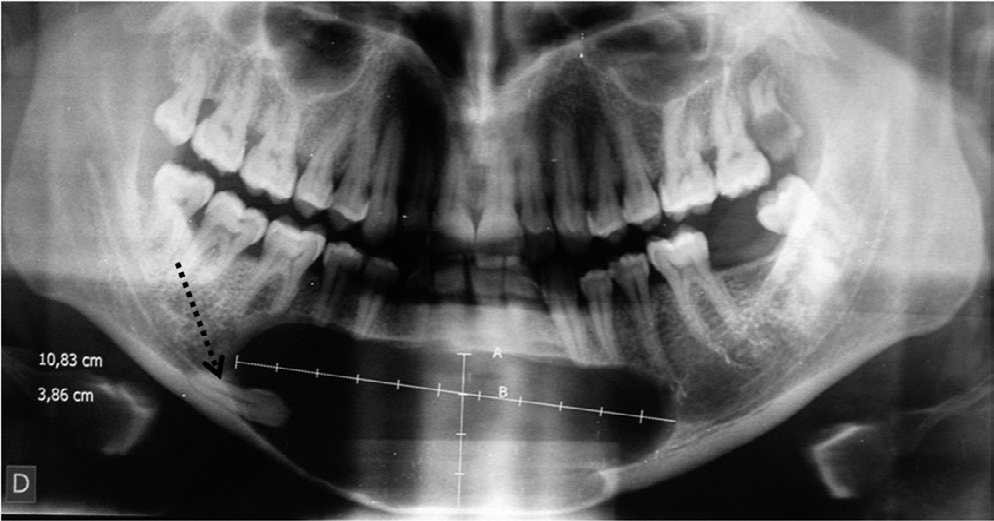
Panoramic radiography showing a cystic image occupying the entire symphyseal region and extending to the right and left parasymphyseal regions, associated with an impacted 43 in horizontal position close to the basilar border
